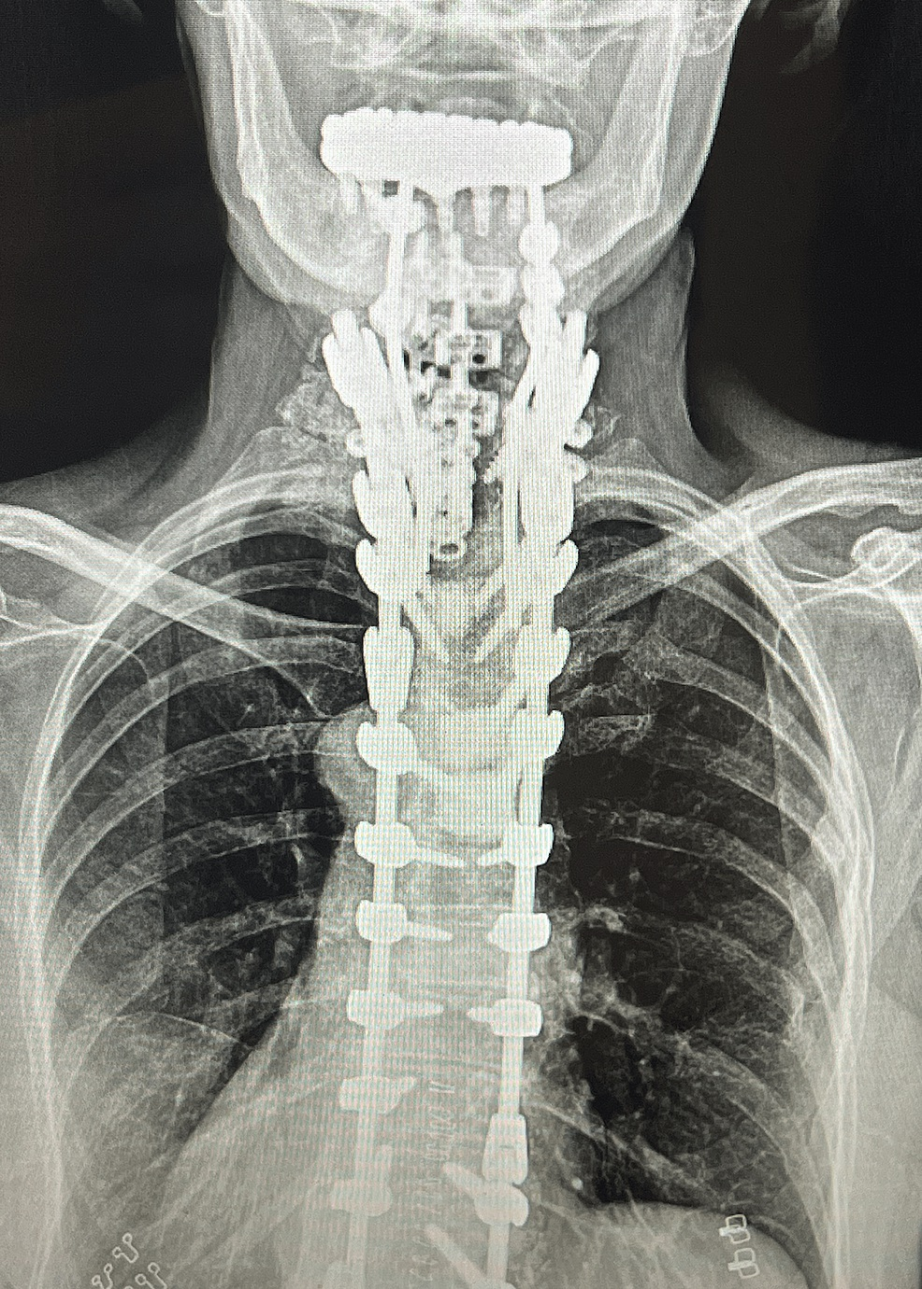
AP postoperative plain film imaging. This is an AP radiograph of the cervical and thoracic spine following the double-plate technique. AP, anterior-posterior
